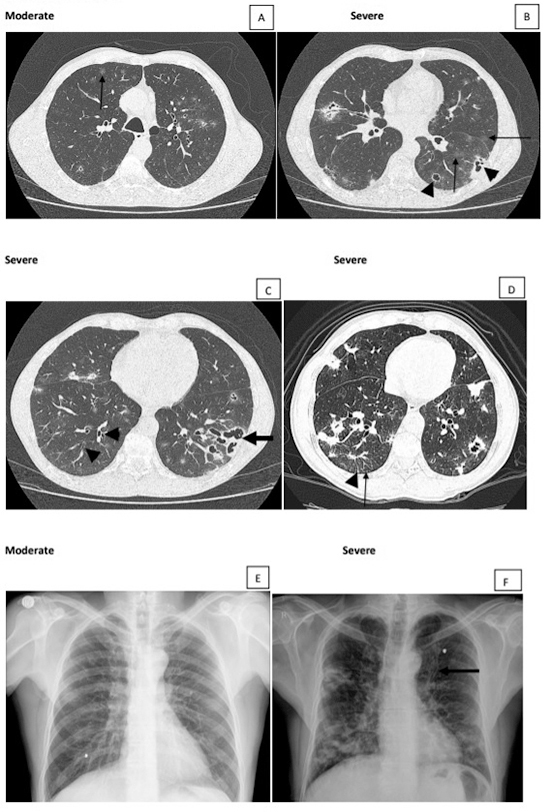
Moderate and severe active lesions. (A) Moderate lesion showing > 3 ground-glass nodules (arrow). (B) Besides ground-glass nodules (arrows), cavitary lesions with and without septations (arrowheads) in greater amount, characterizing severe classification. (C) Bronchiectasis inside consolidation opacity (arrow) and bronchial wall thickening (arrowheads). (D) Focal lesions, ground-glass and intersticial opacities with interlobular septal thickening (arrowhead) and small centrilobular nodules (arrow). (E) Chest radiograph showing only interstitial opacities, a finding in which CT helps to better characterize and classify the lesions. (F) Bronchial wall thickening (tram-track sign) better observed in this patient with severe lesion (arrow).
